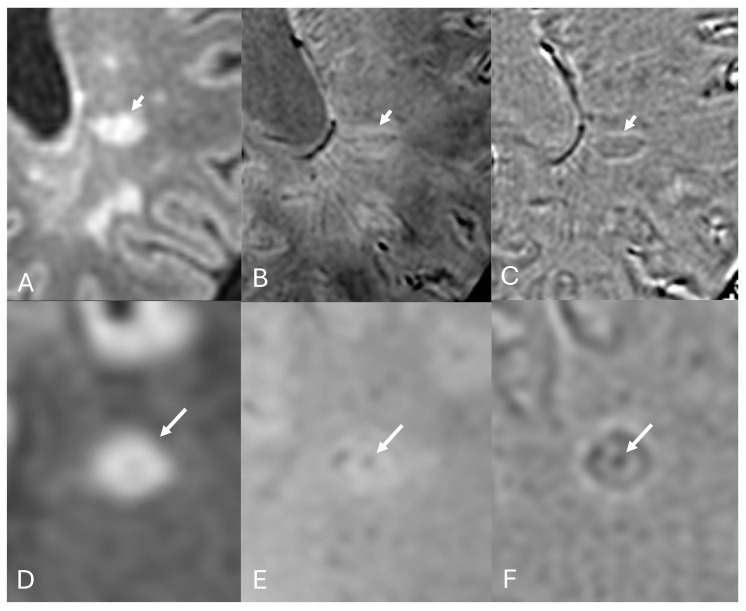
Shapes of MS lesions with central veins. When the MRI plane visualizes the lesion in the longest axis, and the lesion appears with an oval shape in FLAIR (arrow in (A)), the hypointense line of the central vein will be visible in SWI (arrow in (B)) and SWI-phase (arrow in (C)) crossing the center of the lesion and given a coffee-bean appearance. When the lesion appears with a round-shape in FLAIR (arrow in (D)), the central vein appears as a dark point in the center of the lesion in SWI (arrow in (E)), which will frequently have a target or doughnut-shape in SWI-phase (arrow in (F)).
